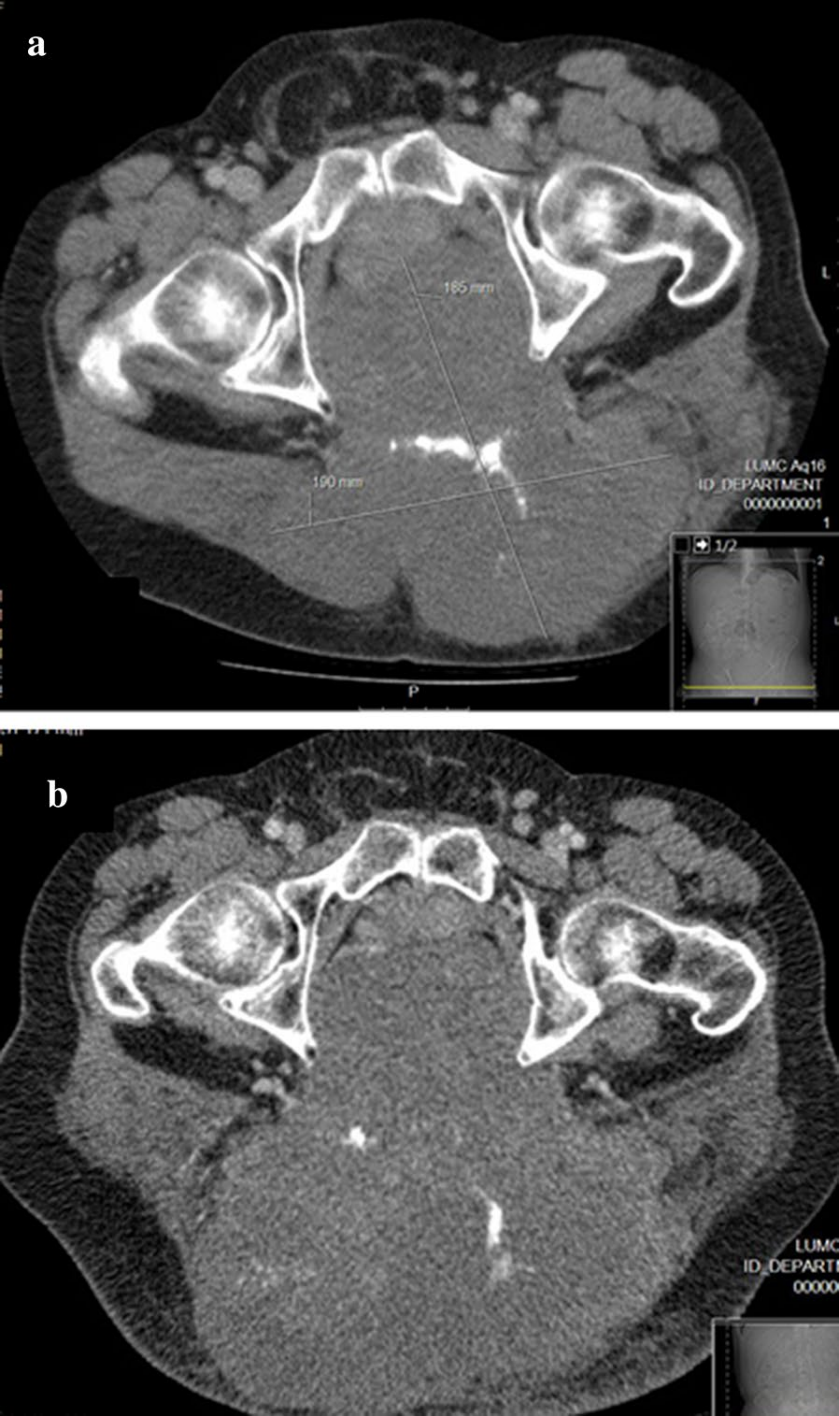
CT images of patient 2 treated with pazopanib. a CT scan at start of pazopanib showing a large sacral chordoma reaching from S2 to coccygis. Measurements: 20 × 16 × 13 cm (AP × LR × CC). b CT scan after 15 months of pazopanib showing progressive disease. Measurements: 21 × 24 × 23 cm (AP × LR × CC)
